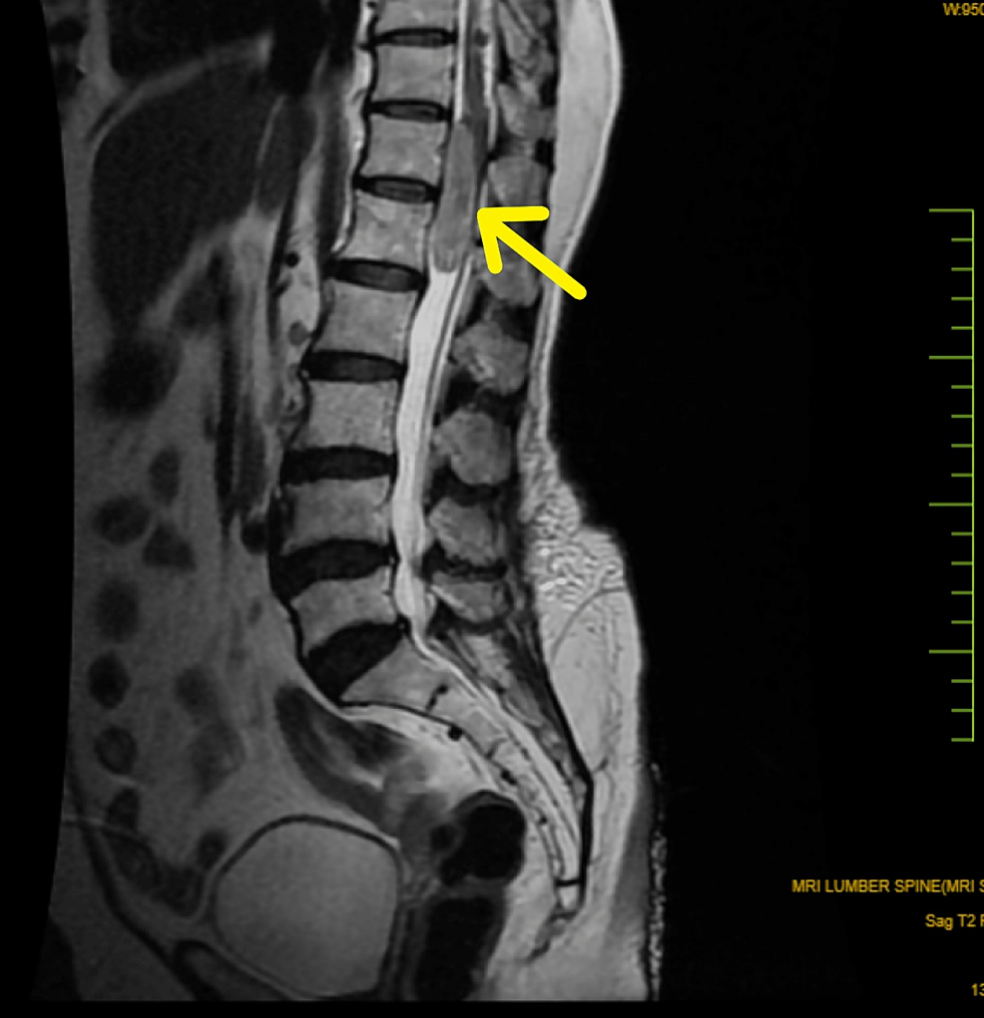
T2-Weighted MRI (sagittal section) The image shows a well-circumscribed enhancing lesion extending from the upper border of T12 to the lower border of L1. Lesion is intradural extramedullary.
